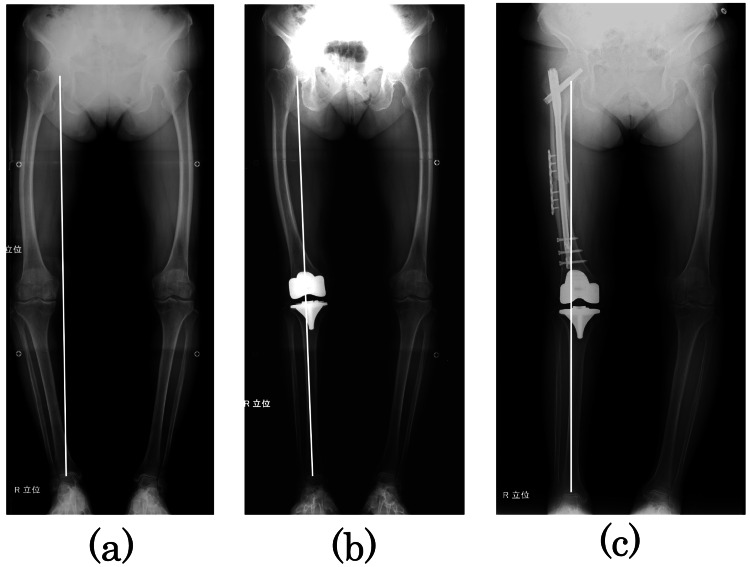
Radiographs of the entire length of the lower limb Radiographs of the entire length of the lower limb: (a) immediately before TKA surgery, (b) immediately after TKA surgery, and (c) immediately after this surgery. The white line shows the Mikulicz line. TKA: total knee arthroplasty
